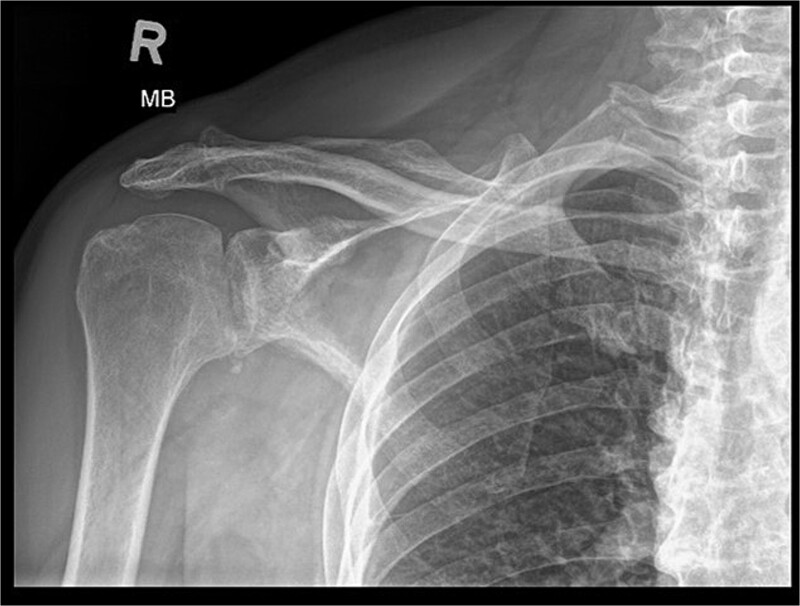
X-ray image before the surgery—AP projection.
